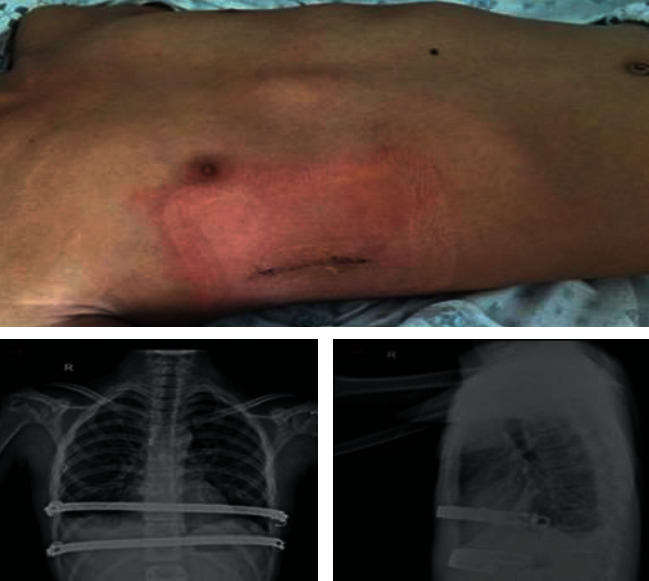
Pectus carinatum comorbid with depressed Harrison groove deformity after surgery (appearance of the chest and anterior and lateral chest X-ray films).
